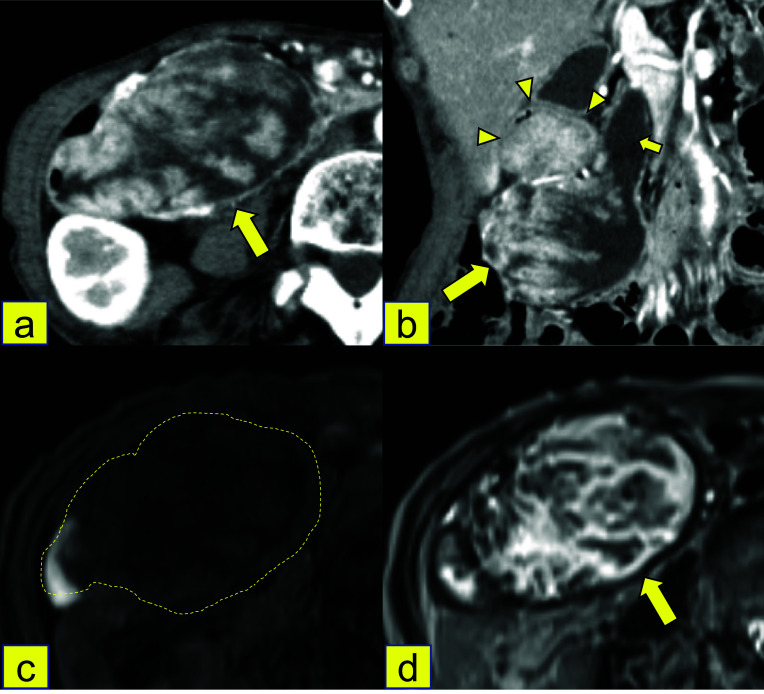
Imaging findings of the patient. a Abdominal contrast-enhanced CT in the axial section showed a 90-mm-sized tumor with a mixture of solid and cystic components with contrast enhancement in the pancreatic head (arrow). b CT imaging of the coronal section showed the tumor (large arrow) grew within the main pancreatic duct (small arrow), not within branches, compressing and narrowing the duodenum (arrowhead). c T1-weighted MRI showed a giant cystic lesion with a clear border and smooth margin in the pancreatic head with low intensity (dotted line). d T2-weighted MRI showed a giant cystic lesion with high intensity (arrow)
